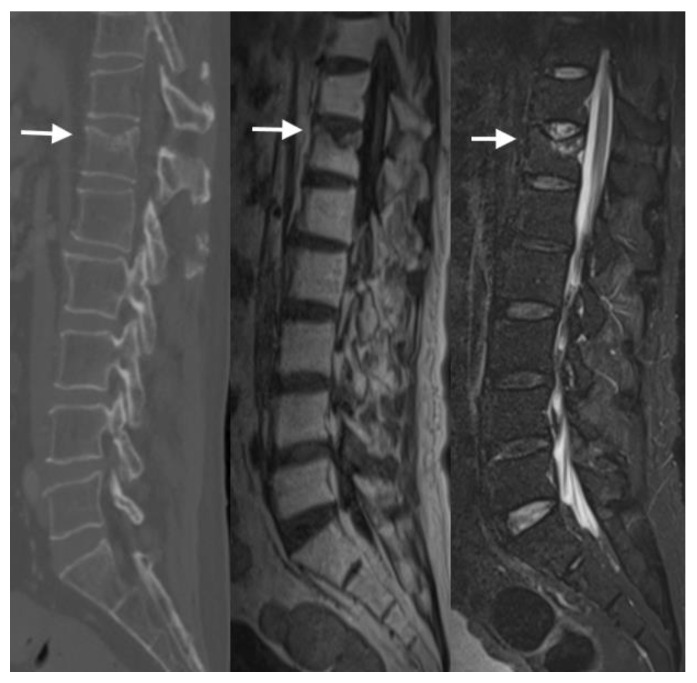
In the sagittal CT (left), T1 MRI (middle), and STIR MRI (right), the arrows show mild chronic superior T12 endplate compression deformity and loss of height with accompanying T1 hypointense/STIR hyperintense enhancing marrow signal abnormality. Slight STIR hyperintensity is seen along the superior T12 vertebral body reflecting edema, which is significantly improved from the prior scan.
